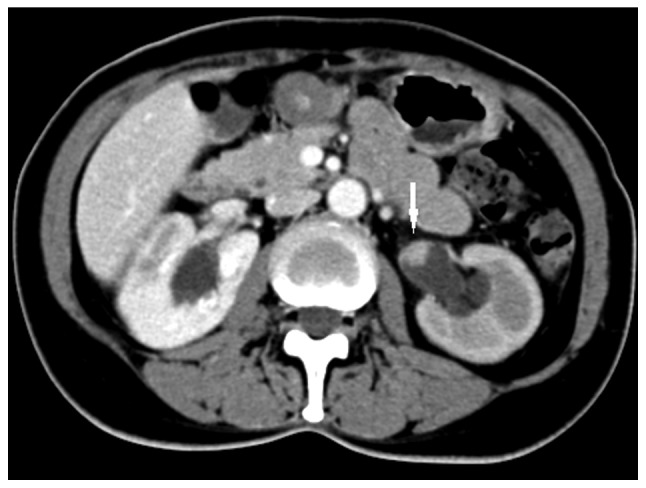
Abdominal computed tomography scan demonstrates a mass in the left renal pelvis.
